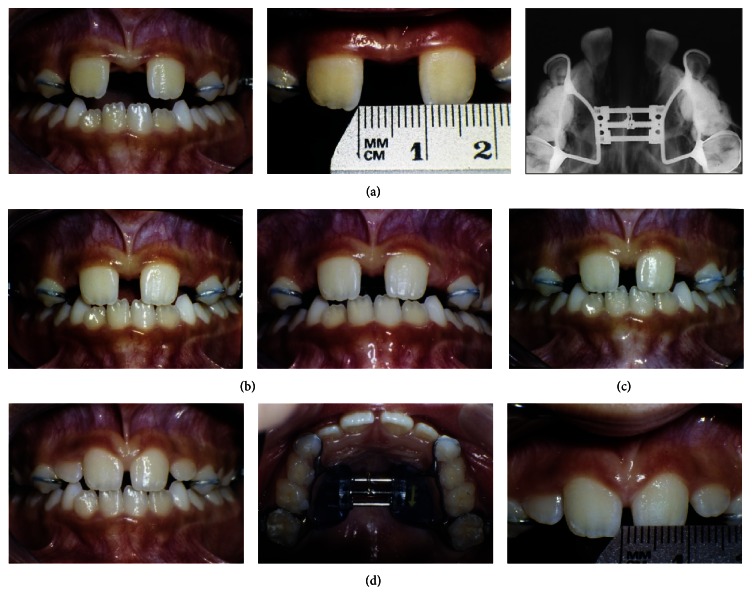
Midline diastema with clinical signs of the suture opening. Occlusal radiographs with real disjunction of the suture. (a) After three weeks and 52 screw activations. (b) 43 days and (c) and (d) 5 months and 6 months after retention. The diastema returned to the initial dimensions in the retention phase of the screw.
